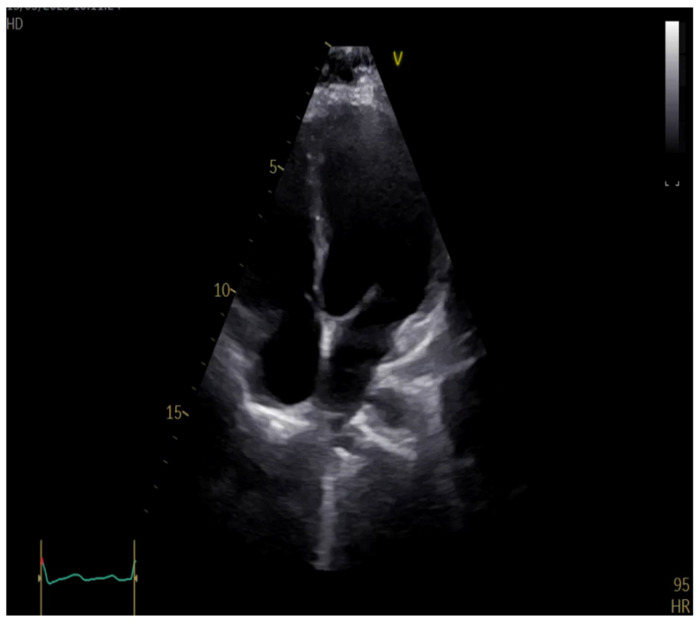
Transthoracic echocardiography at discharge showing reduction in right ventricular size compared with admission, consistent with hemodynamic improvement.
